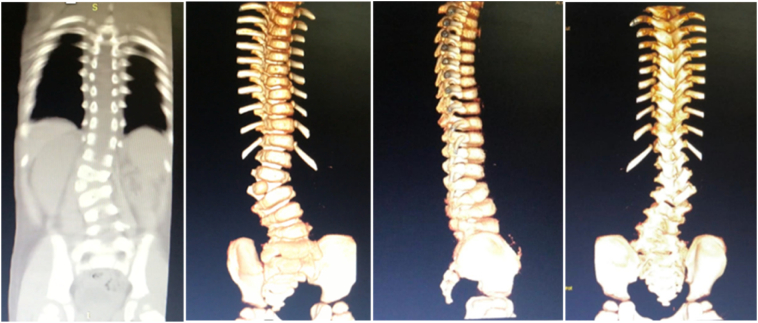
CT-scan and 3D CT-scan of the patient before MSCs implantation. A single fully segmented hemivertebra was visible at L-3 level.
